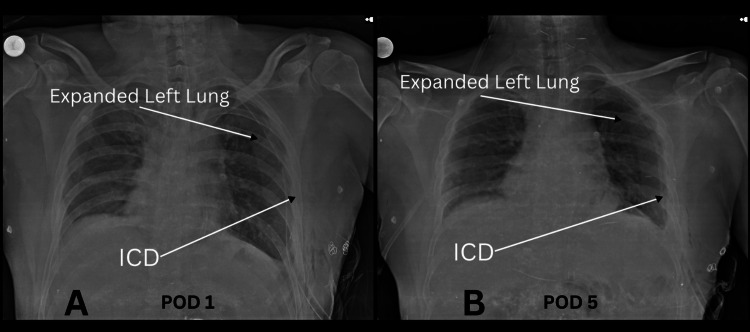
Chest X-ray on A) postoperative day 1; B) postoperative day 5.
